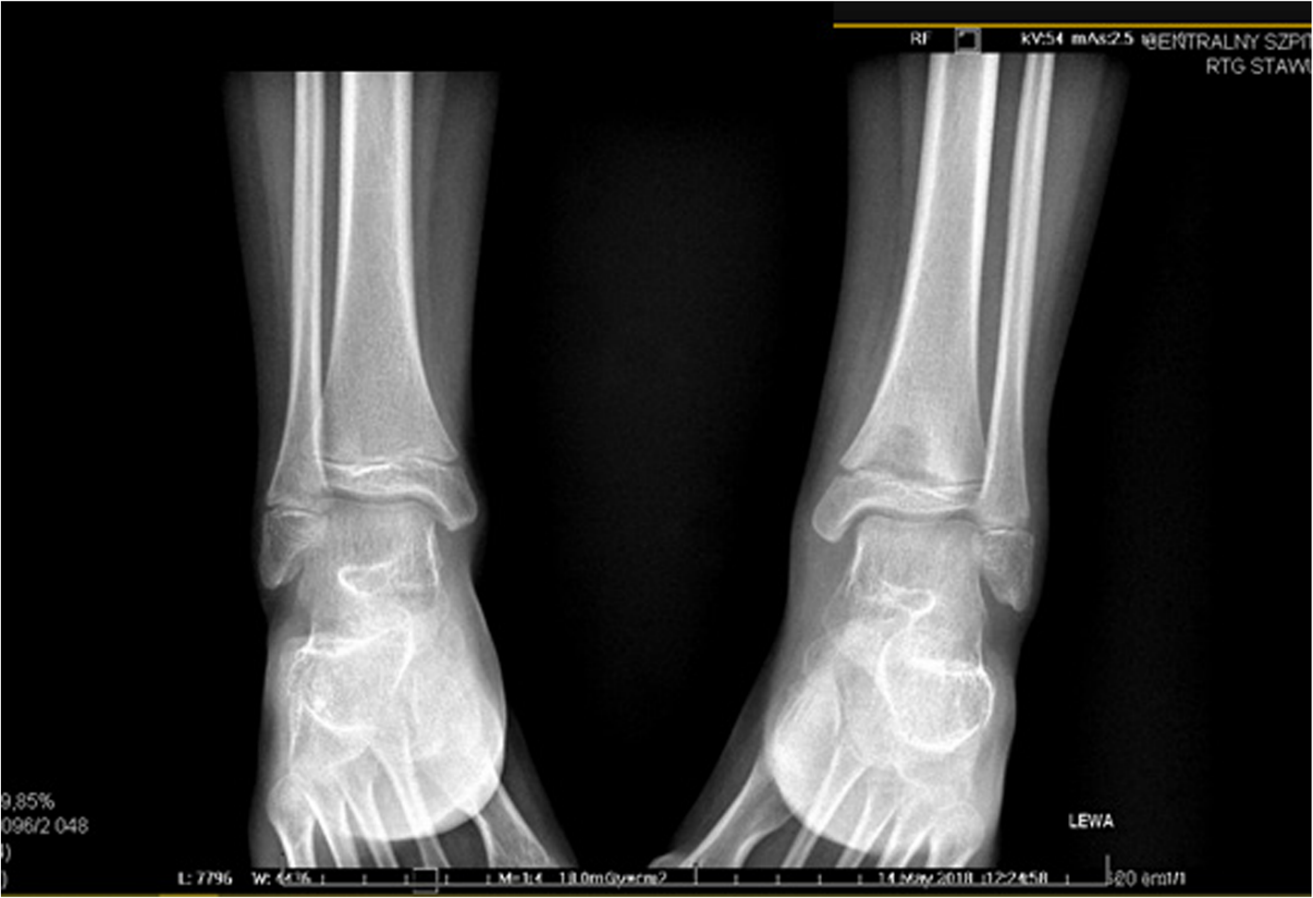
Radiograph showing a large lytic lesion in the metaphysis of left tibia
